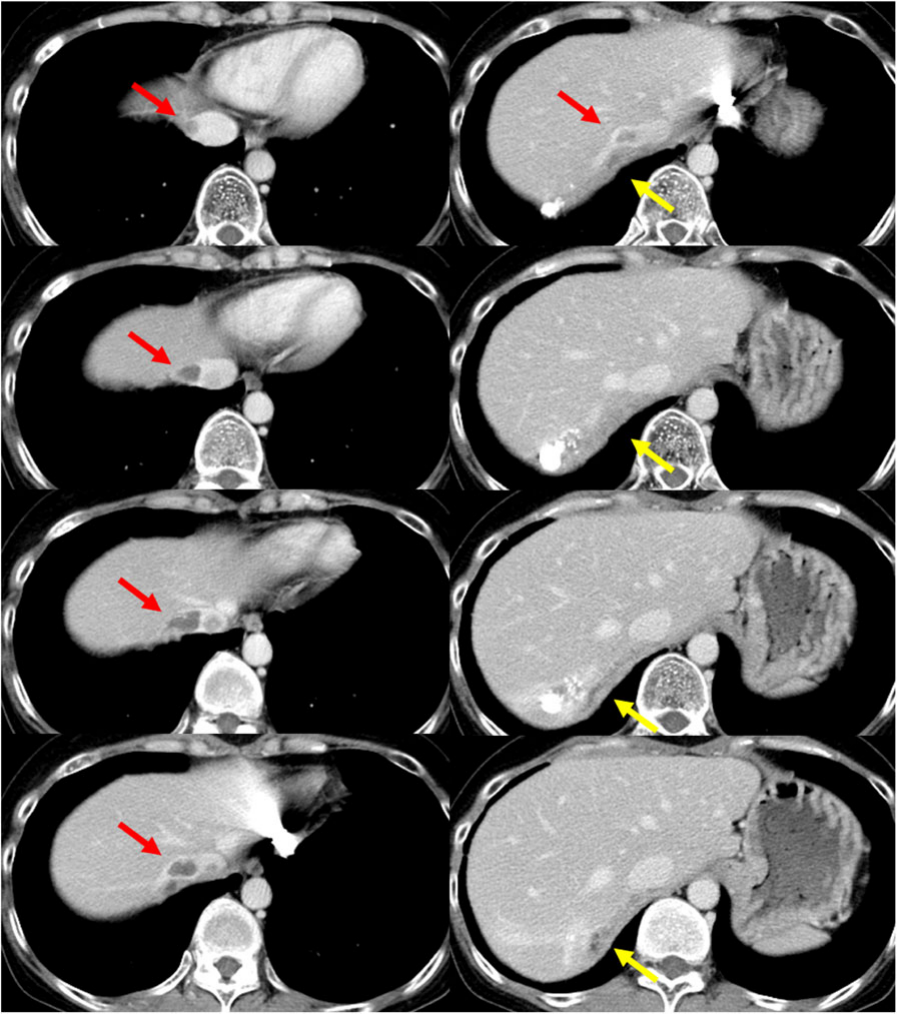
Dynamic-enhanced computed tomography imaging of case 2. A tumor thrombus was detected in the right hepatic vein, inferior vena cava (IVC), and right atrium (RA; red arrow), as well as in the right phrenic vein (yellow arrow)
